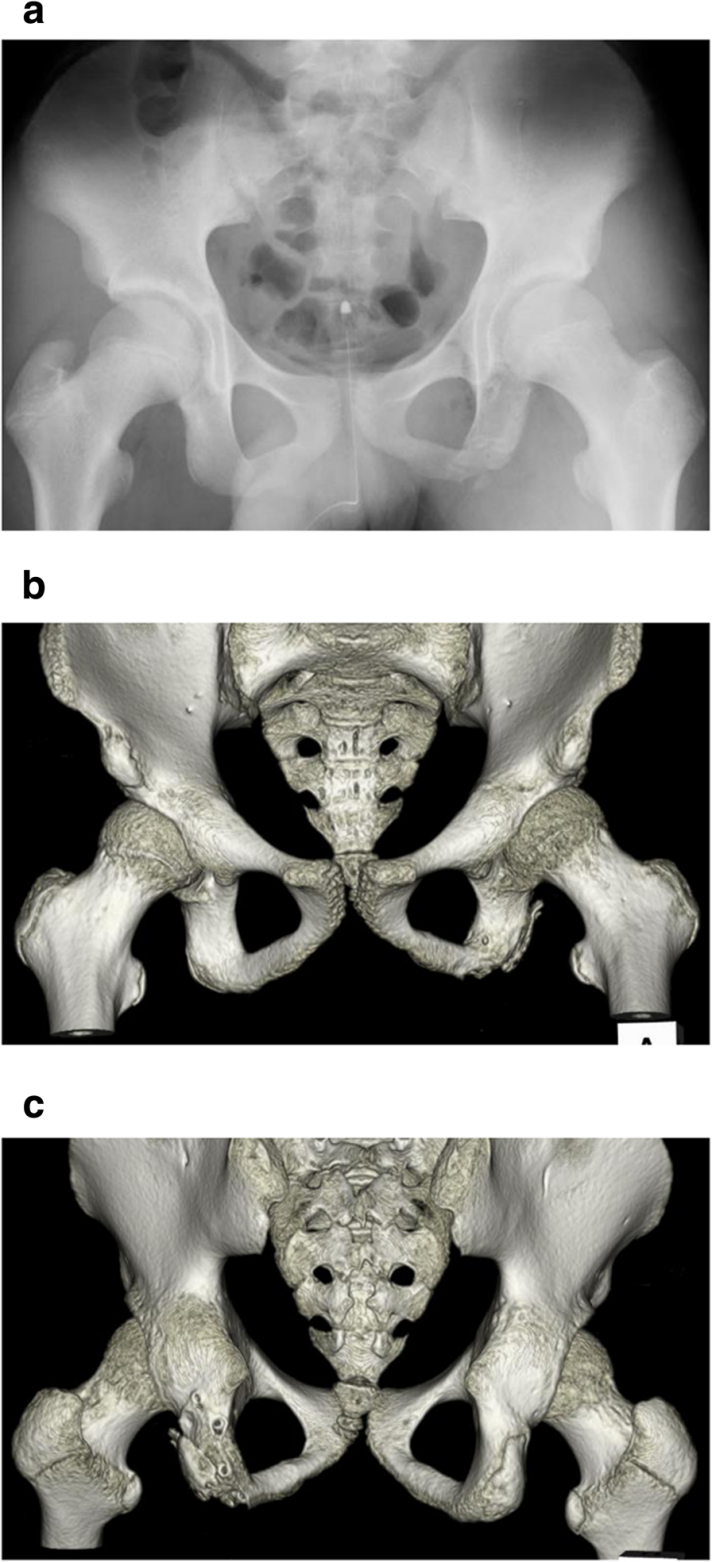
Postoperative imaging of the pelvis. a Plain radiography. b, c Postoperative CT
